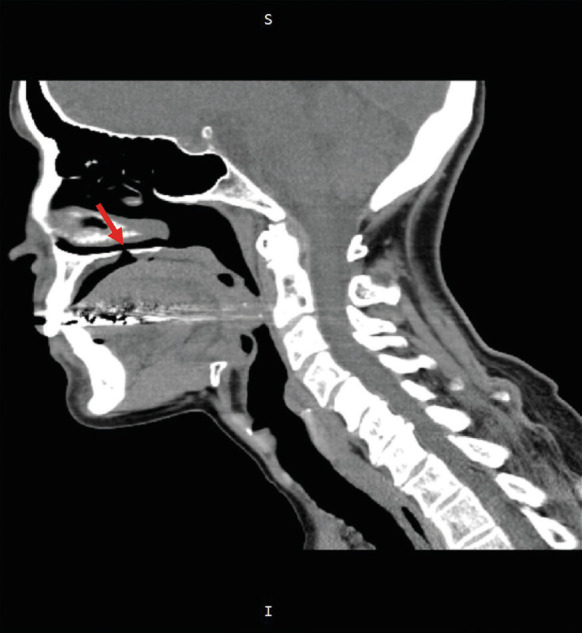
CT scan shows the oroantral communication (arrow). No other pathological features are seen in the oral or nasal mucosa.
